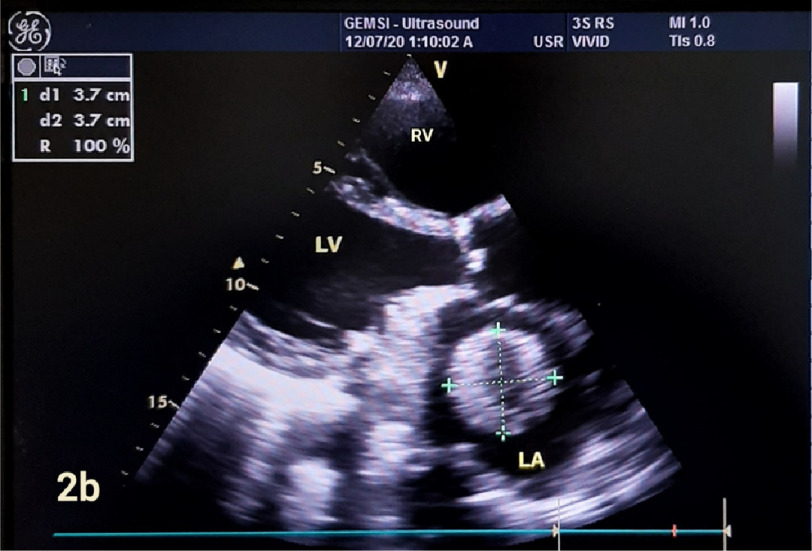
Parasternal long axis view, showing the same ball thrombus with the same dimensions.
